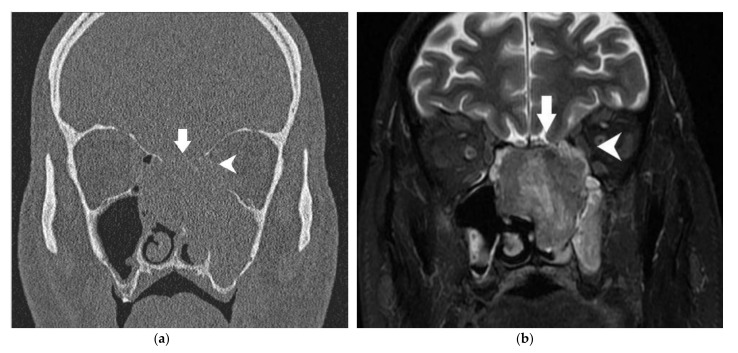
Sixty-three-year-old male subject with left ethmoido-maxillary non-intestinal-type adenocarcinoma. (a) Coronal CT scanner: major erosion of cribriform plate (white arrow) and lamina papyracea (white arrowhead). (b) Coronal MRI (T2 acquisition with fat saturation): white arrow: minor modification of bony skull base; white arrowhead: major modification of lamina papyracea, regular deformation of orbital content, invasion of the fat between tumor and oculomotor muscle. In the radiological conclusion, the bony skull base, dura, cerebral parenchyma, orbital bony walls and orbital content were all considered tumor-free.
